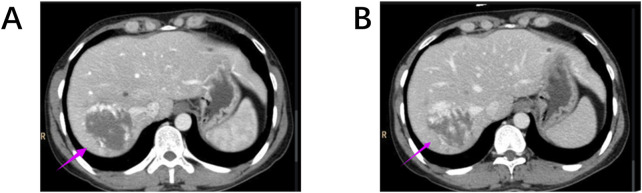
(A, B) Hepatic cavernous hemangiomas also exhibit a “centripetal filling” pattern similar to RH on contrast-enhanced CT scans.
